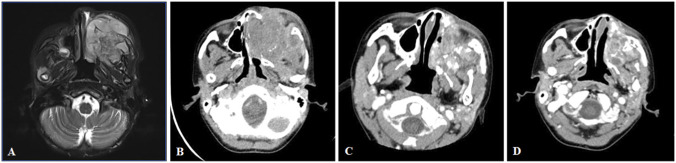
Imaging findings of Patient 1. (A) Maxillofacial MRI acquired in May 2025 shows local tumor recurrence. (B) Contrast-enhanced CT performed in July 2025 after two cycles of salvage ICE chemotherapy showed progressive maxillofacial soft-tissue disease; the lesion measured 63.5 mm × 55.6 mm × 65.7 mm. (C) Follow-up contrast-enhanced CT in October 2025 after two cycles of tislelizumab-containing chemoimmunotherapy showed marked tumor regression, with the lesion reduced to 41.4 mm × 44.6 mm × 41.6 mm. (D) Contrast-enhanced CT in January 2026 after four cycles of combination therapy; the lesion measured 40.6 mm × 30.7 mm × 38.8 mm.
